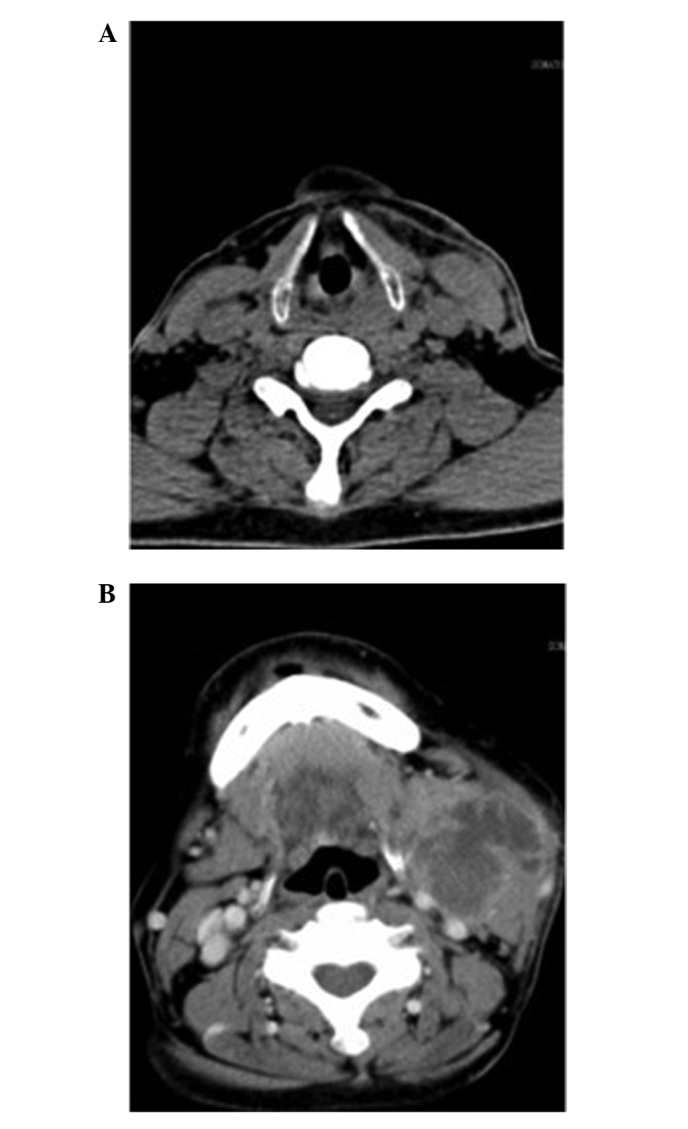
Computed tomography findings of case one. (A) A soft tissue mass was demonstrated in the left pyriform sinus. (B) A 4.4×4.1-cm lesion, which was not well defined from the surrounding healthy soft tissue, was demonstrated on the left side of the neck.
